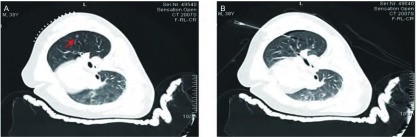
穿刺针定位于肺磨玻璃样微小结节（GGO）病灶的CT扫描图。A：自制定位器和GGO病灶；B：穿刺针定位于病灶。 CT scanograms of the ground glass opacity (GGO) lesions with pilot pin. A: Homemade locator and GGO lesions; B: Lesions with pilot pin.
